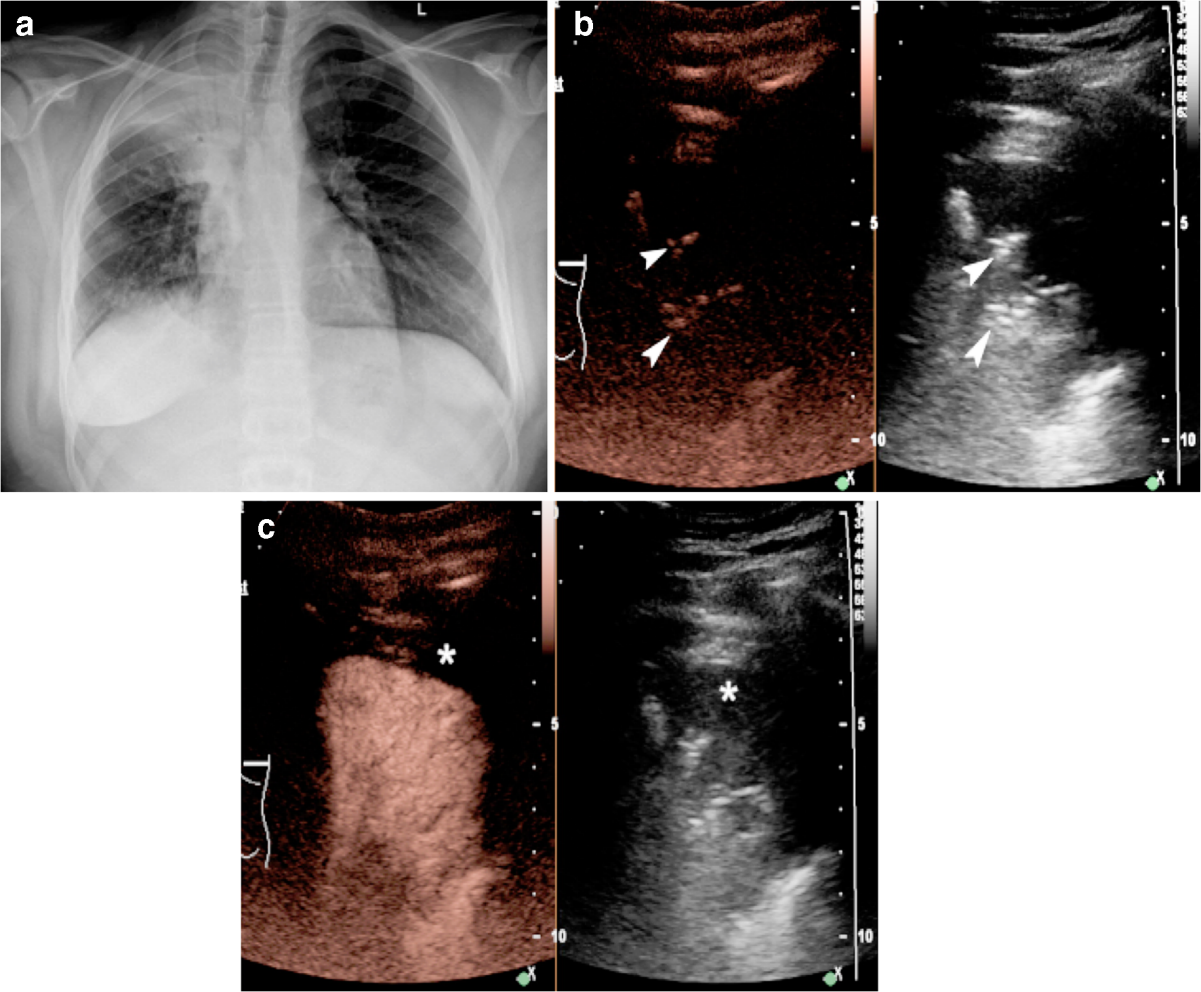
Endocarditis and right-side pneumonia (*Staphylococcus aureus*) in a 14-year-old girl. a Posteroanterior chest radiograph demonstrates right upper lobe consolidation containing branching air-bronchogram. b Baseline pre-contrast US scan in dual-screen mode with simultaneous display of contrast mode (*left*) and gray-scale mode (*right*) before contrast agent injection. Coronal plane. Within the area of lung consolidation, highly echogenic branching structures (*arrowheads*) appear similar in contrast and reference gray-scale images, suggestive of air inside the bronchi (ultrasonographic air-bronchogram sign). c Contrast-enhanced ultrasound (CEUS) following intravenous administration of contrast agent. Dual-screen mode with simultaneous display of contrast mode (*left*) and gray-scale mode (*right*) in coronal plane. The consolidated lung demonstrates uniform enhancement, excluding the diagnosis of necrotizing pneumonia. Note a small anechoic parapneumonic effusion (*asterisk*)
